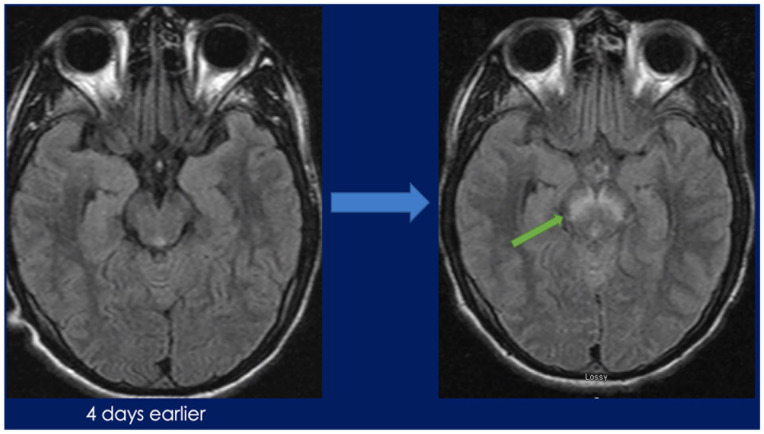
MRI brain at admission (left) and 4 days after admission (right).
